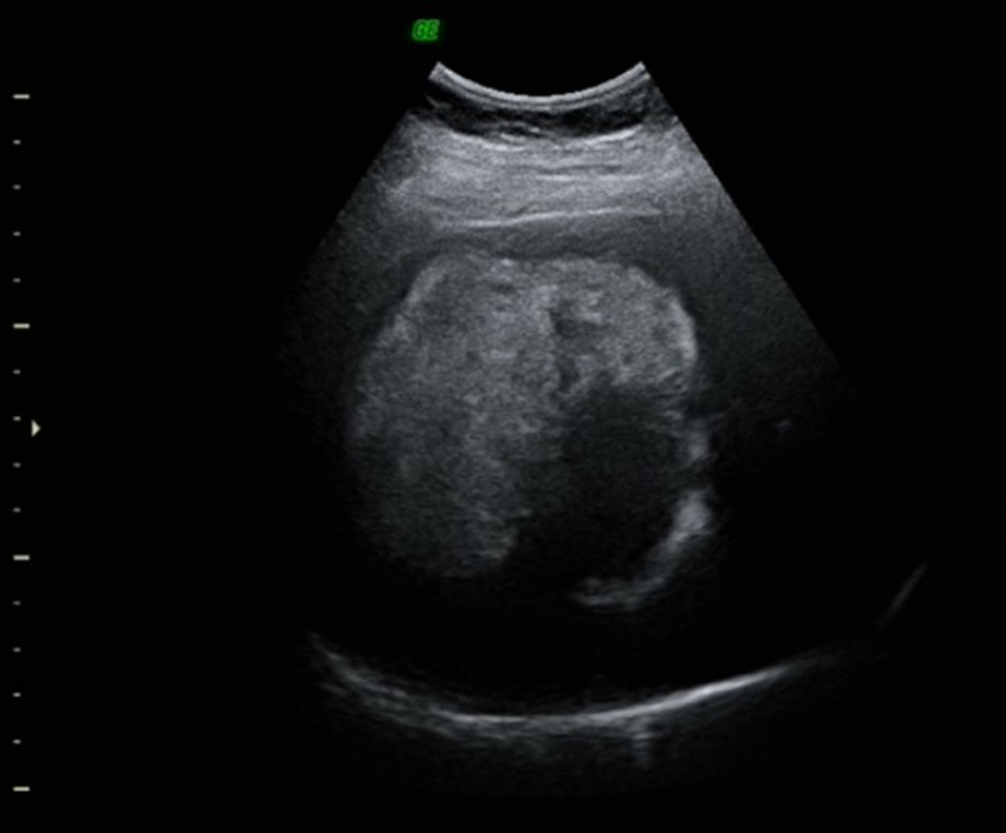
Ultrasound examination: large heterogeneous hyperechoic lesion in the right liver lobe.
